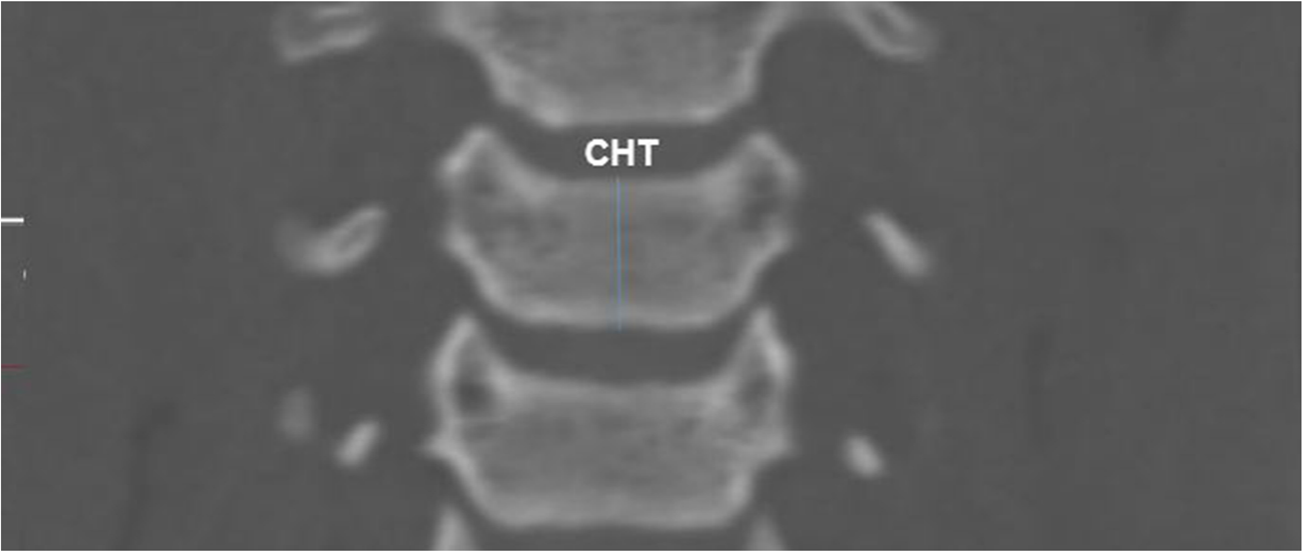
Measurements of CHT on coronal CT image
